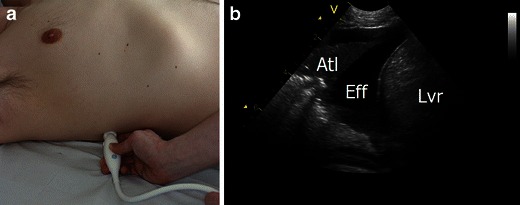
Pleural effusion. a The transducer is placed in a longitudinal axis over an intercostal space on the most dependent area of the chest, since this is the area where free fluid in the chest cavity is expected to be located. b Pleural effusion (*Eff*) often is visualised as a dark, anechoic area lying in between the visceral and parietal pleura on ultrasonography and just above the liver (*Lvr*) or spleen. Underlying compression atelectasis (*Atl*) of the lung is also often present
